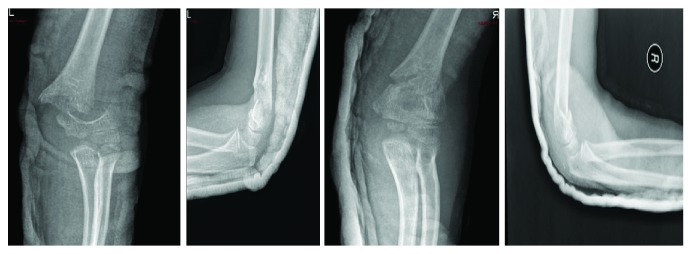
Initial X-rays. AP and lateral views of the right and left elbows.
